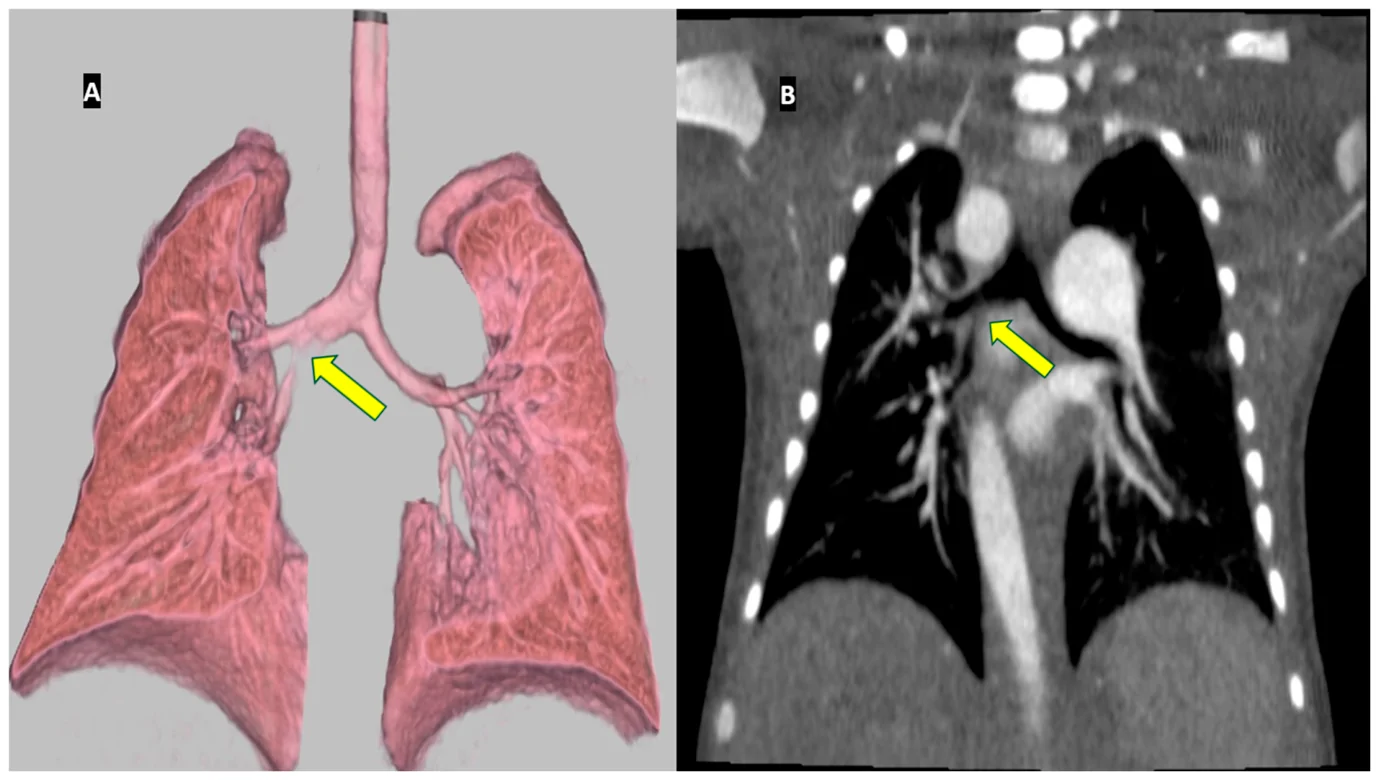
Tracheobronchial compression by the dilated right pulmonary artery in a patient with tetralogy of Fallot with absent pulmonary valve syndrome (TOF-APVS), a hallmark feature of this rare congenital anomaly. (A) Three-dimensional CT reconstruction (volume-rendered image) of the tracheobronchial tree displayed alongside the pulmonary parenchyma, demonstrating extrinsic compression of the right main bronchus by the aneurysmally dilated right pulmonary artery (arrow). (B) Contrast-enhanced coronal CT image showing the spatial relationship between the dilated right pulmonary artery and the right main bronchus, with severe luminal narrowing extending to the level of the bronchus intermedius (arrow).
